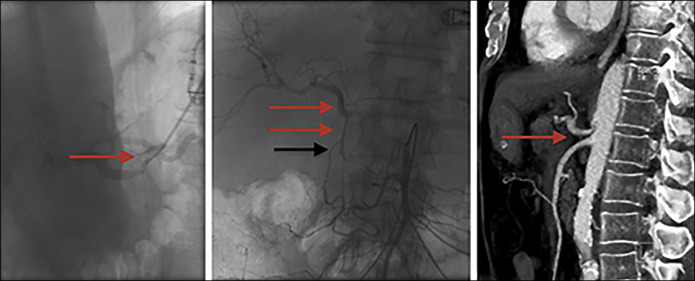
Lateral view selective catheter angiography (left image) confirming a tight stenosis of the celiac trunk origin (arrow). Neutral view catheter angiography of the superior mesenteric artery (middle image) depicting retrograde filling of the gastroduodenal artery and hepatic artery (black arrow). Also note a small pseudoaneurysm in the distal part of the gastroduodenal artery (dotted arrow). Celiac trunk stenosis (red arrow) depicted in CT angiography sagittal reformat (right image).
